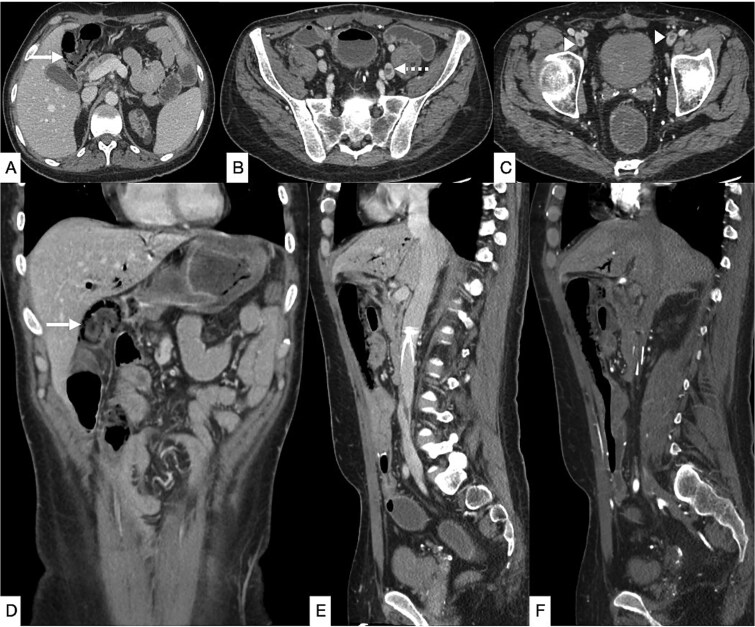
Contrast-enhanced CT of the abdomen and pelvis in axial (A–C), coronal (D), and sagittal (E, F) planes showing diffuse pneumatosis intestinalis just proximal to the previous anastomotic site (bold arrow), consistent with bowel ischemia. New thrombi are identified within the left common iliac vein (dashed arrow) and bilateral common femoral veins (arrowhead). Mild residual pneumobilia is present. An IVC filter is seen *in situ*.
